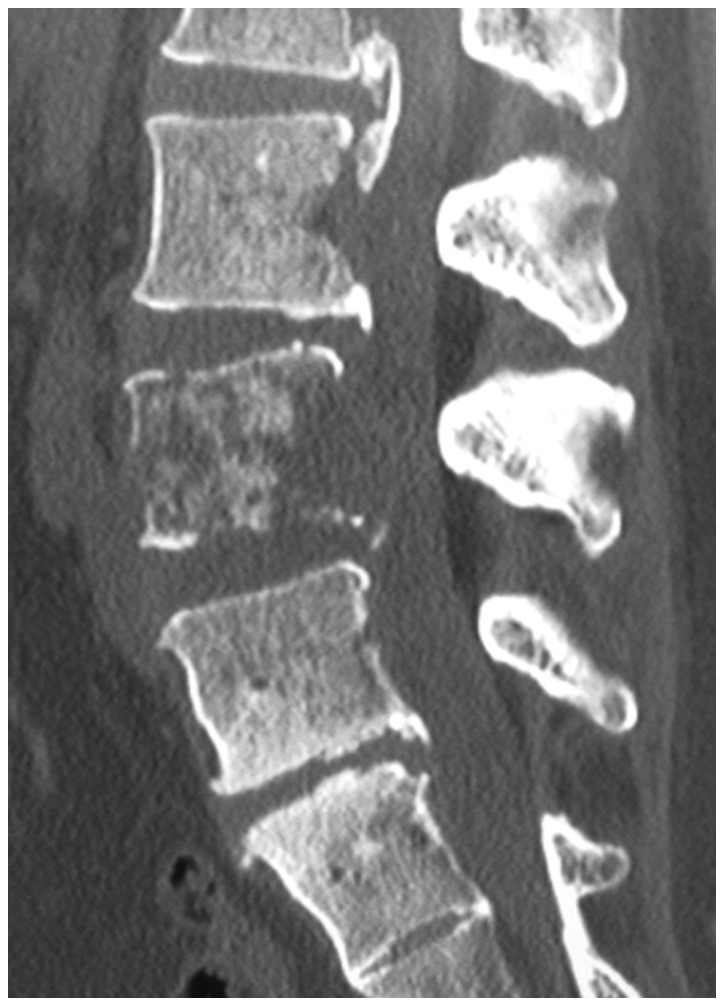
Computed tomography at the level of L4 reveals osteolytic destruction of the L4 vertebra with a defect on the posterior wall of the vertebra in the sagittal view.
